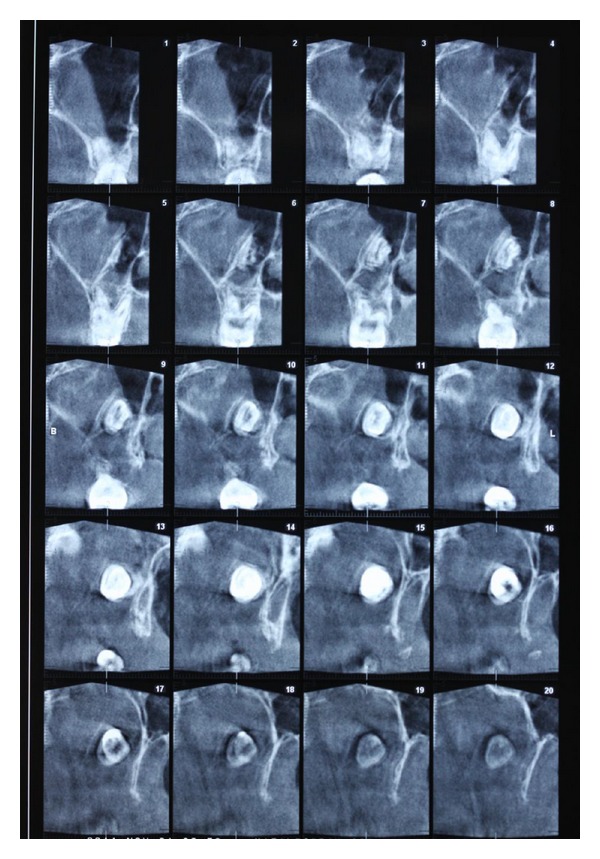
CT revealed that the tooth was located superiorly between the distal margin of maxillary tuberosity and the anterior border of lateral pterygoid plate.
